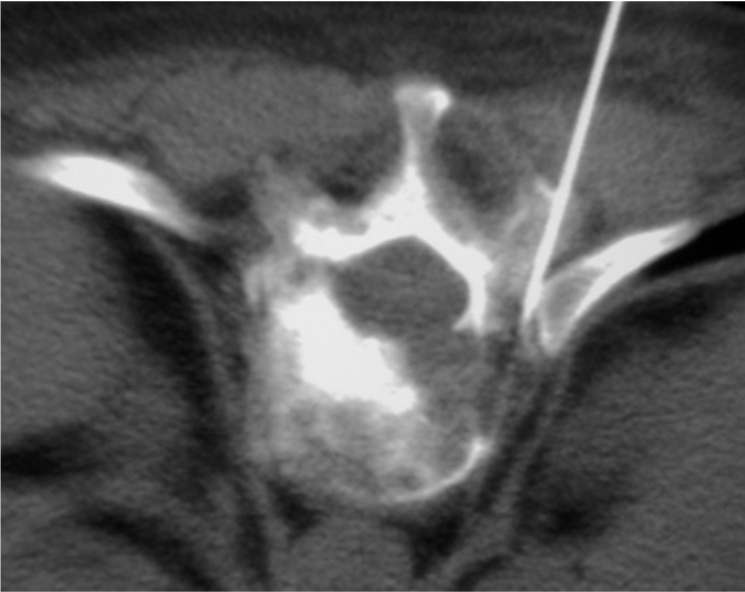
Biopsy of the T12 vertebra using the transcostovertebral approach in a 40-year-old woman with breast carcinoma. CT fluoroscopic image taken with the patient lying prone shows the tip of the Ostycut needle passing through the costovertebral junction towards the right T12 vertebral body osteolytic lesion.
